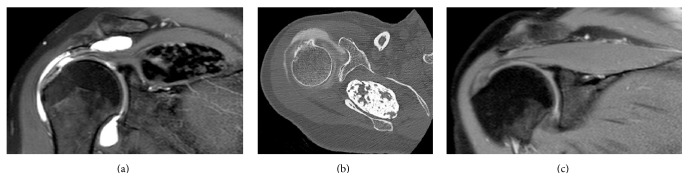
Radiological evaluation of supraspinatus muscle and tendon. MRA showed a calcified mass, as well as a supraspinatus tendon thinning (a). Preoperative CT was performed for a better definition of the volume of the well-delimited calcified mass into supraspinatus muscle (b). Five years ago, a supraspinatus muscle MRI excluded intramuscular mass on this time (c).
